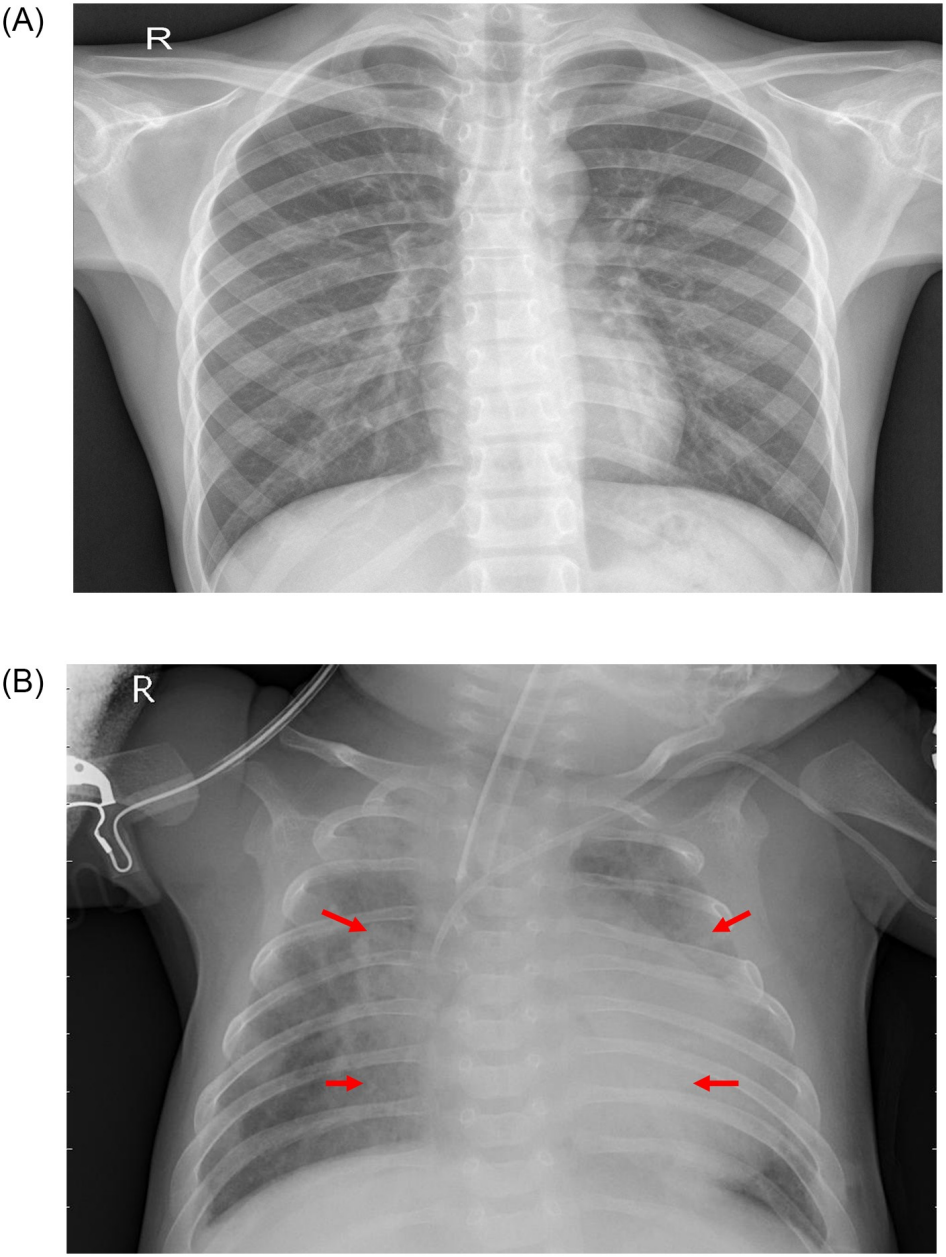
Examples of two X-ray plates that display (a) a healthy lung and (b) a pneumonic lung. The red arrows in (b) indicate white infiltrates, a distinguishing feature of pneumonia. The images were taken from the Kermany dataset [4].
